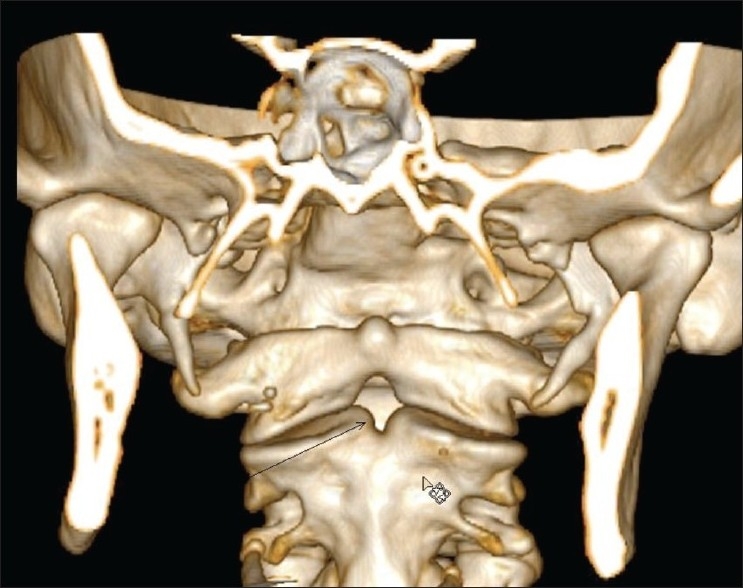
Three-dimensional volume-rendered post-processed CT scan shows absence of the odontoid process (arrow)
